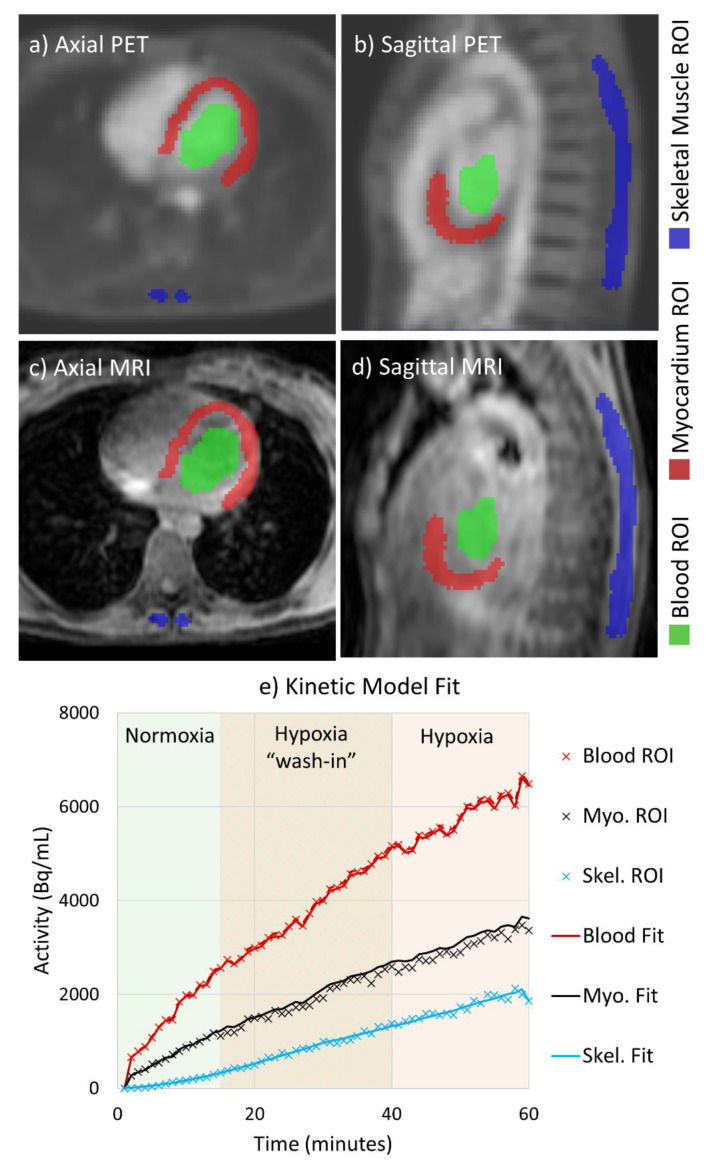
PET image processing method. Regions of interest (ROIs) were draw on the left ventricular (LV) myocardium, the LV cavity, and the paraspinal muscles using temporally averaged PET images (a,b) and co-registered MRI images (c,d). (e) the measured time-activity curves for each ROI (x’s on the graph) were used to fit 3-compartment models of FDG in the myocardium and skeletal muscle, in order to calculate the myocardial metabolic rate of glucose (MMRglc) and skeletal muscle metabolic rate of glucose (SkMRglc). The amount of F-18 fitted to each compartment over time is plotted as solid lines.
